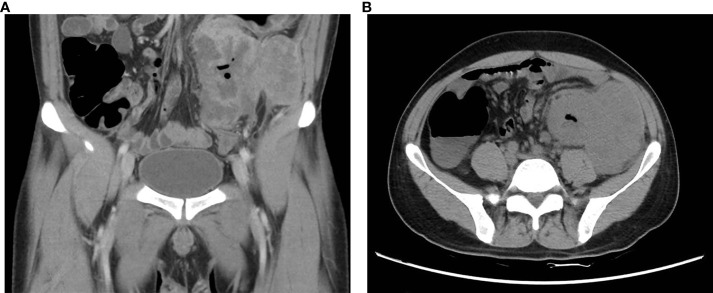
Preoperative CT images of this patient. (A) Coronal section CT showed blurred boundaries between the descending colon mass and the adjacent peritoneum and abdominal wall, as well as blurred periintestinal fat spaces (yellow arrow); (B) Transverse plane CT revealed irregular thickening of the intestinal wall of the descending colon (yellow arrow), forming soft tissue masses approximately 9.9*8.5*10.4 cm.
